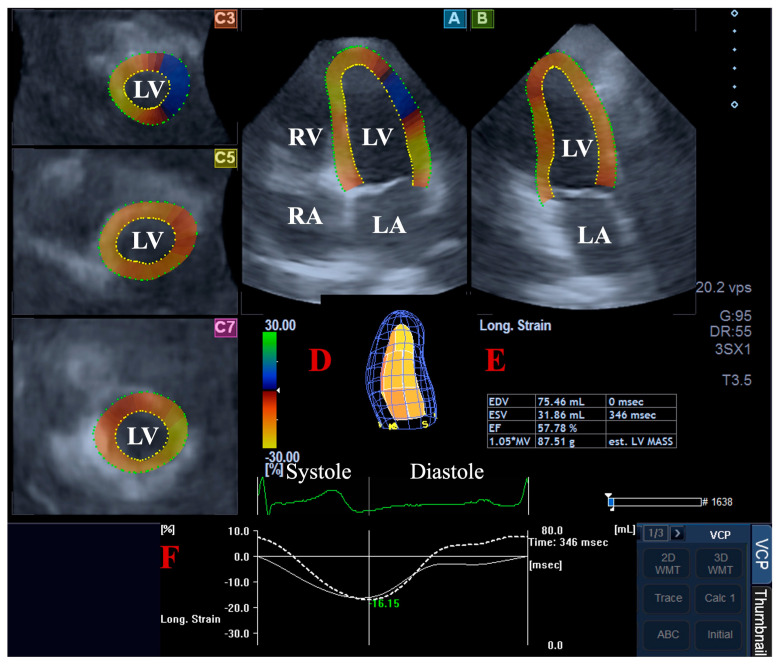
Volumetric assessment of the left ventricle (LV) by three-dimensional (3D) speckle-tracking echocardiography: apical four-chamber (A) and two-chamber long-axis views (B) and short-axis views at basal (C3), midventricular (C5), and apical LV levels (C7) are shown together with a virtual 3D model of the LV (D) and calculated LV volumes (E). Time—global LV circumferential strain curve (white line) and time—LV volume changes curve (dashed white line) are demonstrated as well (F). Abbreviations: LA, left atrium; LV, left ventricle; RA, right atrium; RV, right ventricle; EDV, end-diastolic volume; ESV, end-systolic volume; EF, ejection fraction.
